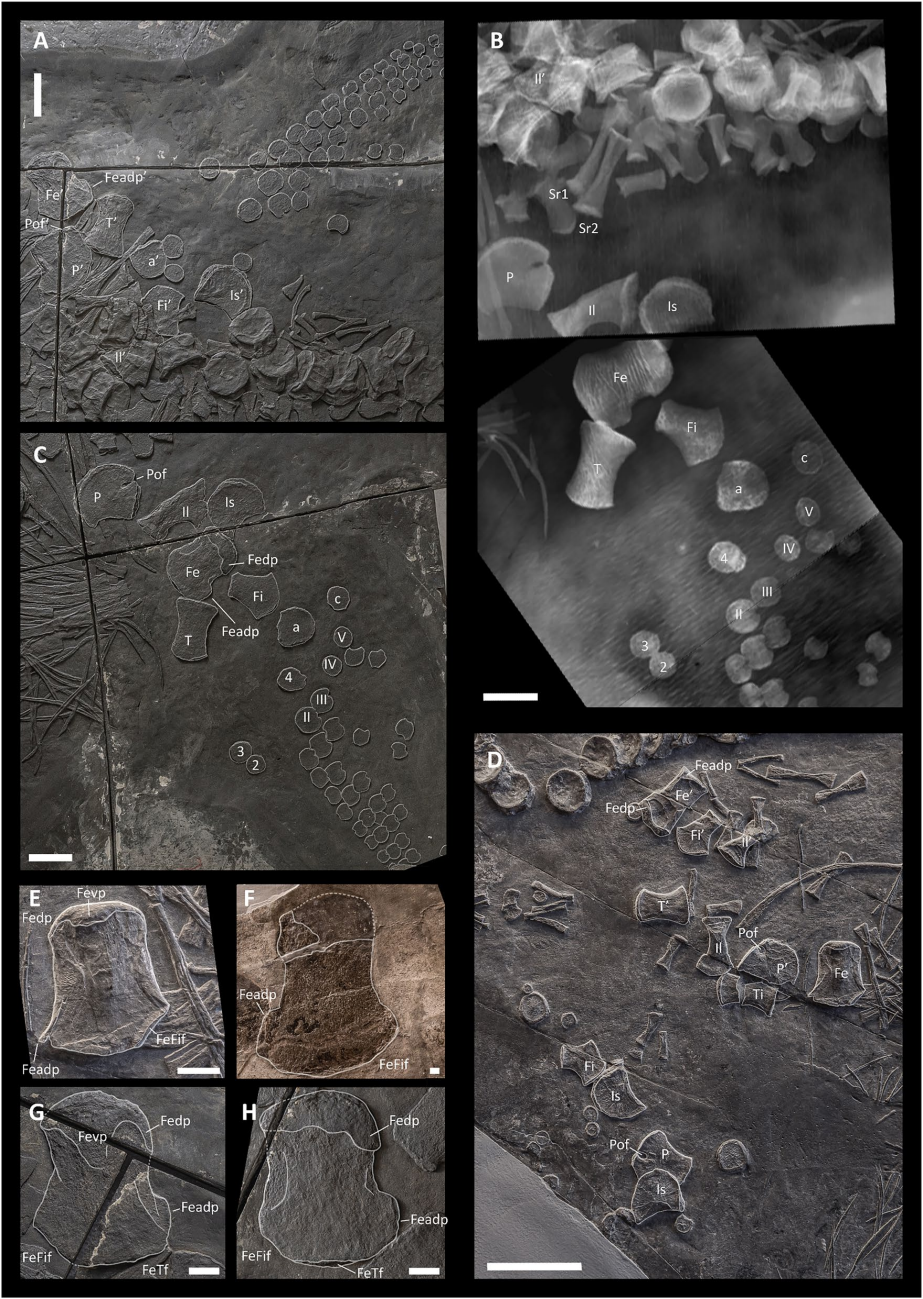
Left (A) and right (C) hindfin and pelvic girdle elements of BES SC 999; (B) CT scan rendering of the right pelvic girdle and forefin elements of BES SC 999; (D) hindfin and pelvic girdle elements of PIMUZ T 4376; (E) right femur of PIMUZ T 4376; (F) femur of PIMUZ T 4847; (G, H) left and right femora of BES SC 999. *a* astragalus, *c* calcaneum, *Fe* femur, *Feadp* femur anterodistal process, *Fedp* dorsal process of the proximal epiphysis of the femur, *FeFif* femoral facet for the fibula, *FeTf* femoral facet for the tibia, *Fevp* ventral process of the proximal epiphysis of the femur, *Fi* fibula, *Il* ilium, *Is* ischium, *P* pubis, *Pof* obturator foramen of the pubis, *Sr* sacral rib, *T* tibia; 2, 3, and 4, distal tarsals; II, III, IV, and V, metatarsals. The apostrophe (‘) always indicates a left element. Scale bars represent 5 cm in A–D, and 1 cm in E–H
